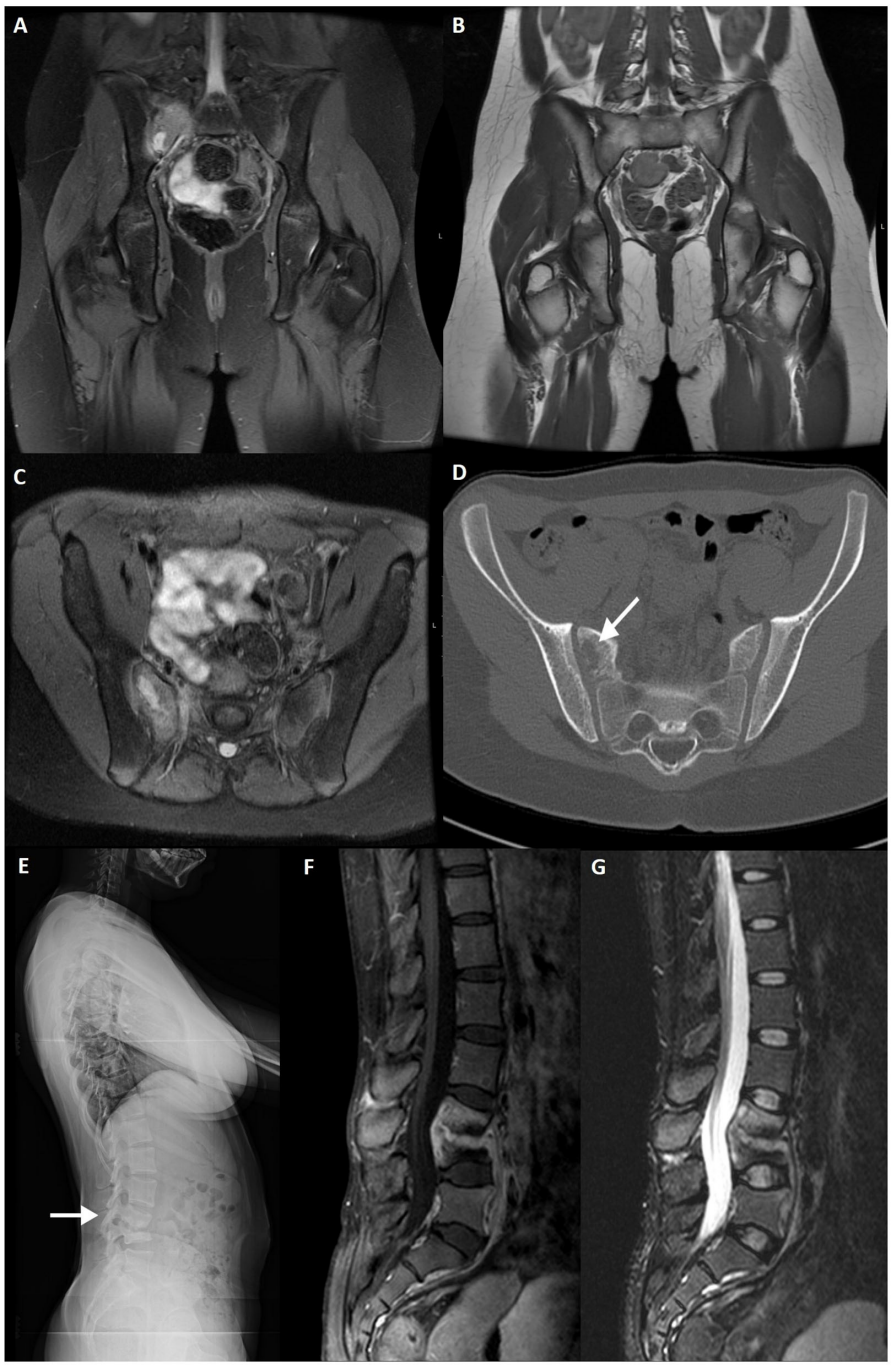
Fat-saturated PD (A) and T1WI (B) coronal slices, fat-saturated PD (C) axial and CT axial slices (D) of a 12-year-old female patient with proven *Streptococcus* spp. osteomyelitis of the right sacrum (arrow). A lytic lesion is seen adjacent to the right sacroiliac joint. Lateral lumbar radiography (E), post-contrast fat-saturated T1WI (F) and TIRM (G) sagittal slices of a 26-year-old female patient with confirmed tuberculous spondylodiscitis of L3–L4 segment (arrow).
